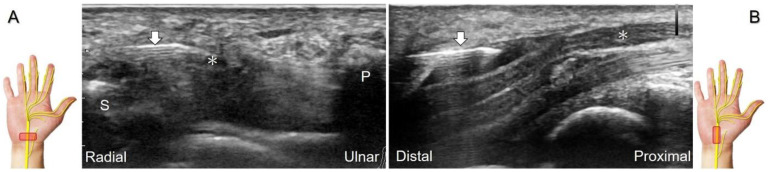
Ultrasound-guided hydrodissection of the median nerve under short-axis (A) or long-axis (B) imaging. Asterisk: median nerve; arrows: needle. S: scaphoid; P: pisiform.
